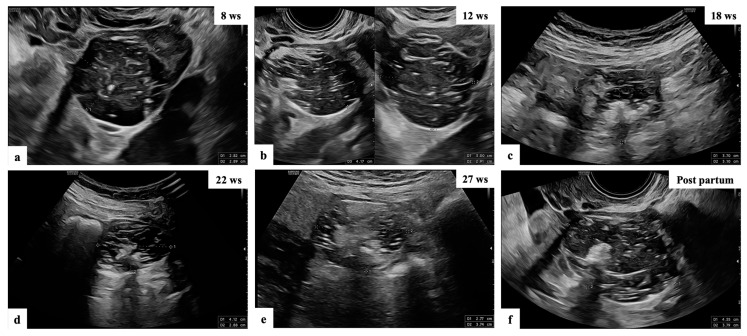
Ultrasound follow-up of patient n° 10 (a–f): grayscale ultrasound images showing adnexal masses with mixed content suspected of teratomas, as reported by the experienced examiner in the original ultrasound reports.
